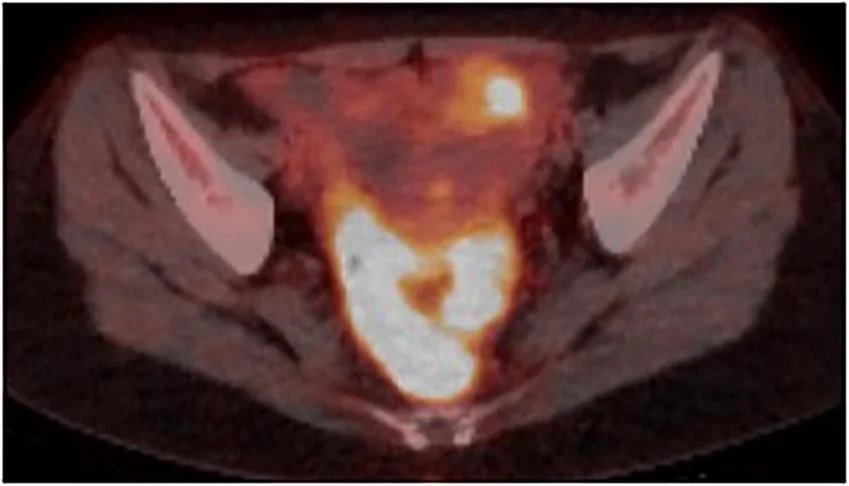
PET FDG CT scan after 4 cycles of treatment showing a new non-specific avid lesion in the left hemi-pelvis.
